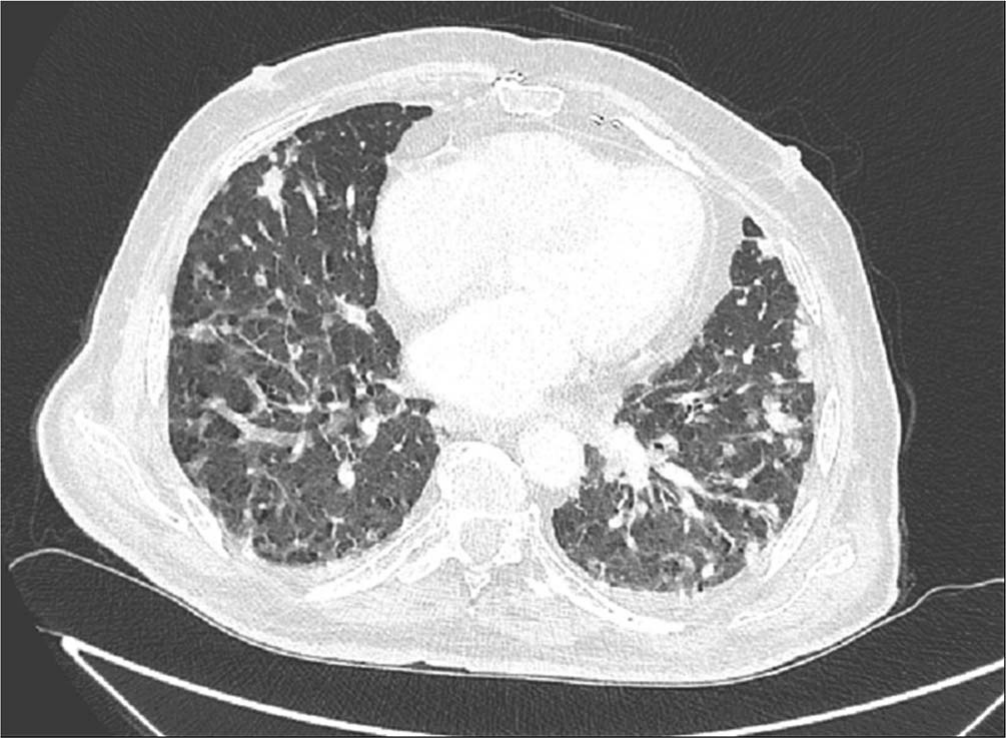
Chest CT revealed multiple pulmonary nodules.
